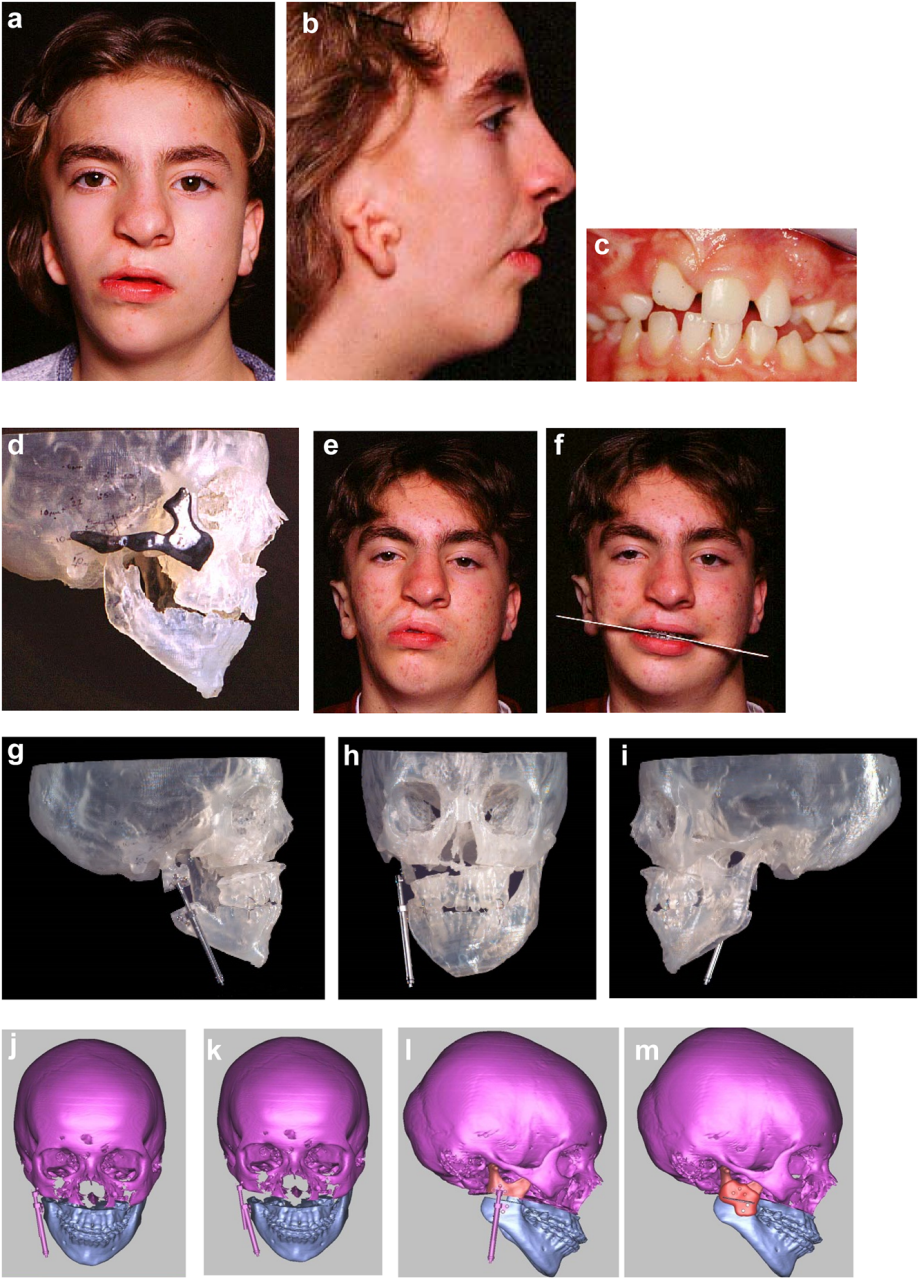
Planning for bimaxillary DO. (a–c) 12 year old male with PK IIa HFM and a repaired right sided UCLP. (d–f) Guide for zygomatic augmentation with rib graft and measurements for bone anchored ear prosthesis with outcome of both. (g–i) Plan on SLA model from CT scan. The maxillary attachment at the nasal septum is retained and the left maxilla rotates upwards during distraction for which bone needs to be removed. (j–m) Virtual bimaxillary distraction plan in another patient.
